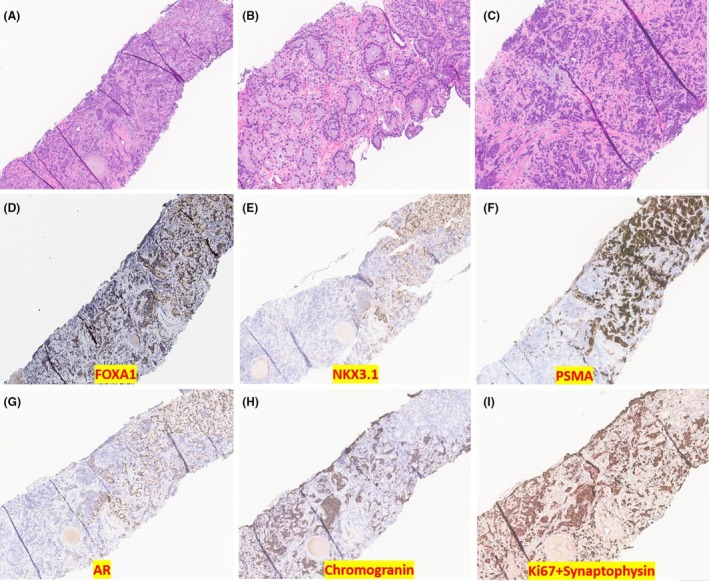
FOXA1 expression in a primary de novo small cell carcinoma of the prostate. (A–C) This prostate core biopsy shows mixed prostatic adenocarcinoma (B) and small cell carcinoma (C, D) Both prostatic adenocarcinoma and small cell carcinoma show diffuse expression of FOXA1; however, the small cell carcinoma component loses expression of traditional prostatic markers including NKX3.1, PSMA, AR (E–G), while expressing neuroendocrine markers including synaptophysin and chromogranin with a high Ki67 index (H, I). [Colour figure can be viewed at wileyonlinelibrary.com]
